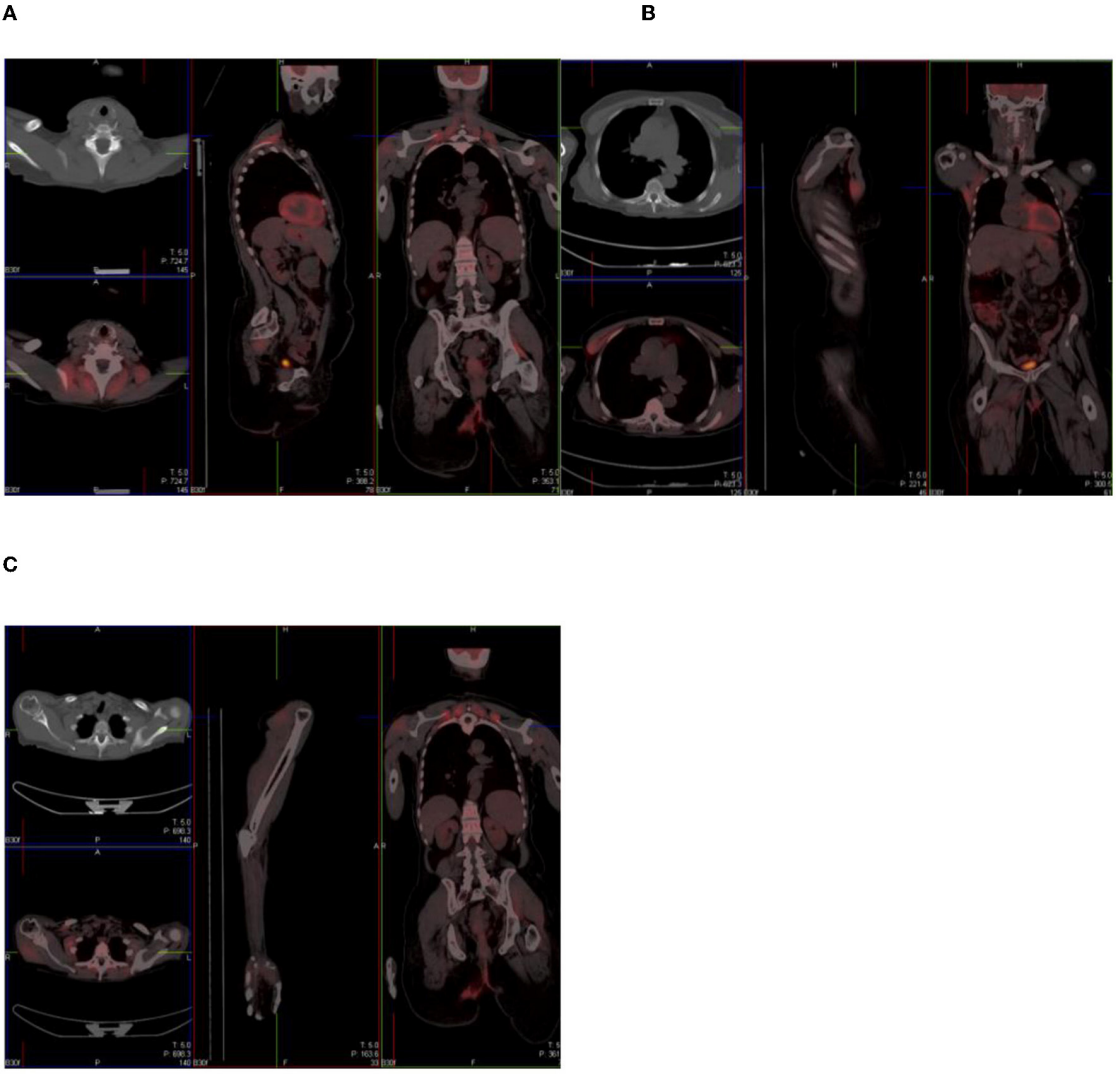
Combined PET/CT images of a 67-year-old woman with anal carcinoma. There is diffuse uptake in the skeletal muscles of the neck (A), chest (B), and upper limbs (C)—related to muscular exertion.
